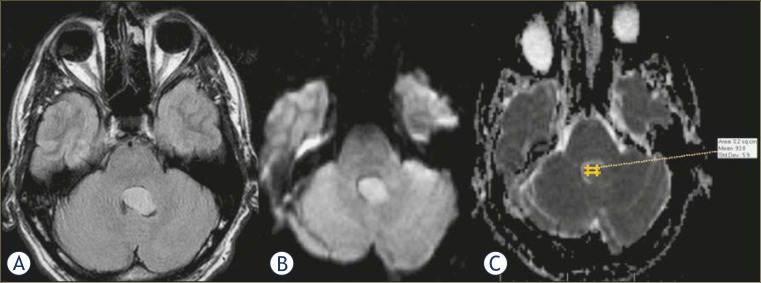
Axial MRI of a patient with grade II ependymoma shows morphologically similar tumour to the one seen in Figure 1A, with moderate hyperintensity in FLAIR image. Diffusion-weighted image and ADC map reveal relatively restricted diffusion, with coefficient of 93.8 × 10^−5^ mm^2^/s.
